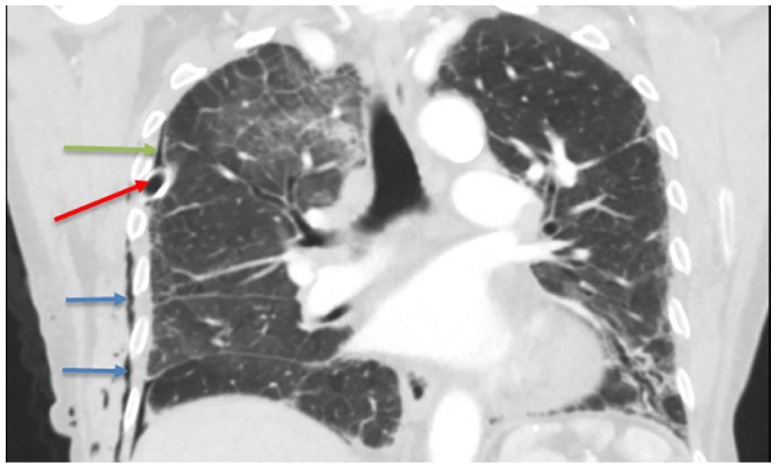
Noncontrast chest CT scan showing resolving right side pneumothorax (green arrow), mild right subcutaneous emphysema (blue arrows), right-side chest tube (placed for tension pneumothorax; Red arrow). No pulmonary nodules were seen.
